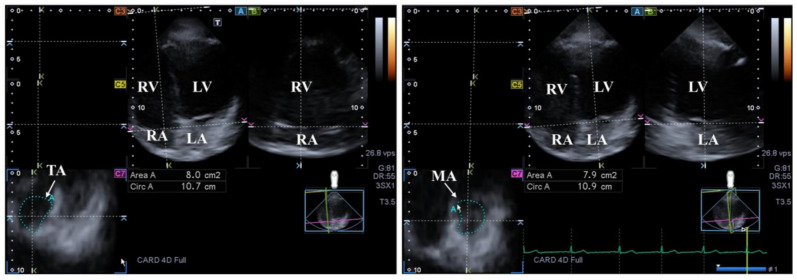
Examination of the tricuspid (TA) and mitral (MA) annuli by three-dimensional (3D) echocardiography (left panel and right panel, respectively). (A) Apical 4-chamber and (B) two-chamber longitudinal views help visualization of valvular annuli on (C7) cross-sectional view. TA and MA planes are marked by a white arrow. TA and MA can be easily detected alongside the heart chambers including the left ventricle (LV) and atrium (LA) and the right ventricle (RV) and atrium (RA).
